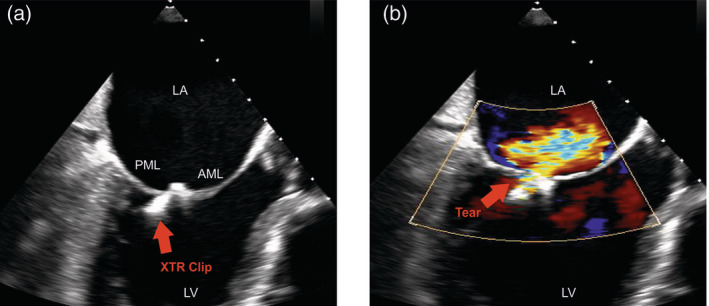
Acute leaflet injury. (A,B) show transesophageal echocardiographic images in one case of acute leaflet tear after implantation of the MitraClip XTR device. The MitraClip XTR device is tilted towards the anterior mitral leaflet (AML) due to tear (B, red arrow) of the posterior mitral leaflet (PML) causing eccentric MR. Left ventricle (LV), left atrium (LA), AML, and PML are labeled accordingly
